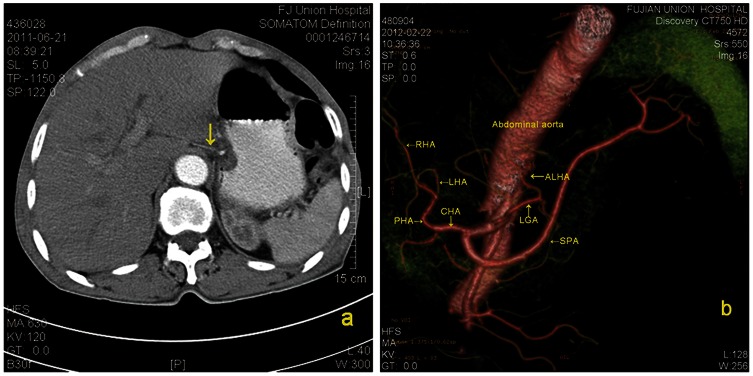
Preoperative enhanced transverse CT image showing a fine accessory left hepatic artery (ALHA) in the gastrohepatic ligament. (a). Three-dimensional CT reconstruction,showing an ALHA originating from the left gastric artery. (b). LGA, left gastric artery; SPA, splenic artery; CHA, common hepatic artery; PHA, proper hepatic artery; LHA, left hepatic artery; RHA, right hepatic artery.
